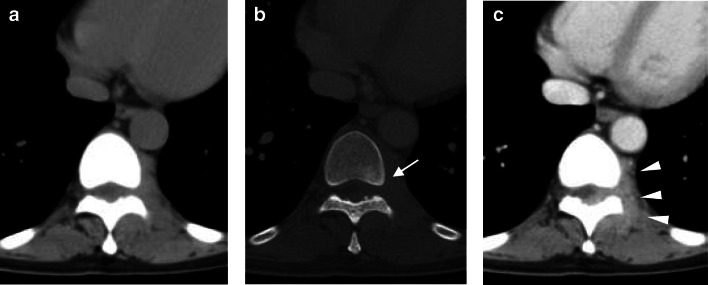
Thoracic spine CT: (a) Soft tissue window. (b) Bone window. (c) Contrast-enhanced CT. Paravertebral mass lesion was observed on the left of the Th10 vertebra. The area adjacent to the tumor-like mass in vertebral body slightly showed bone sclerosis (*white arrow*). The mass lesion and the left side of epidural space and paravertebral muscle were uniformly enhanced. All enhanced area appeared to be connected (*white arrow head*)
